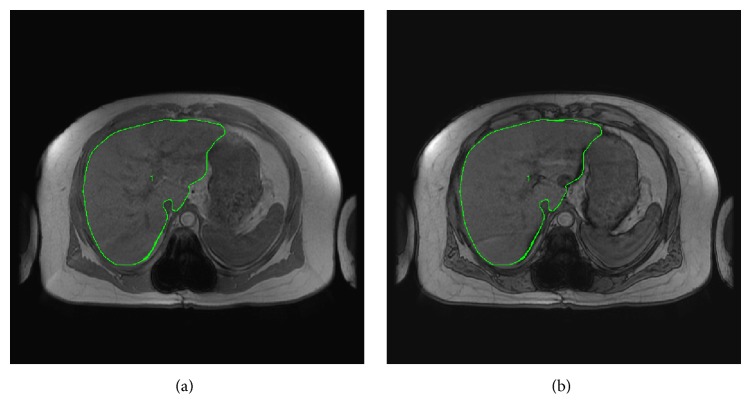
MRI images were used to trace the liver using the in-phase (IP) image (a) and its identical out-phase (OP) image (b) for calculation of the hepatic fat signal fraction (FSF).
